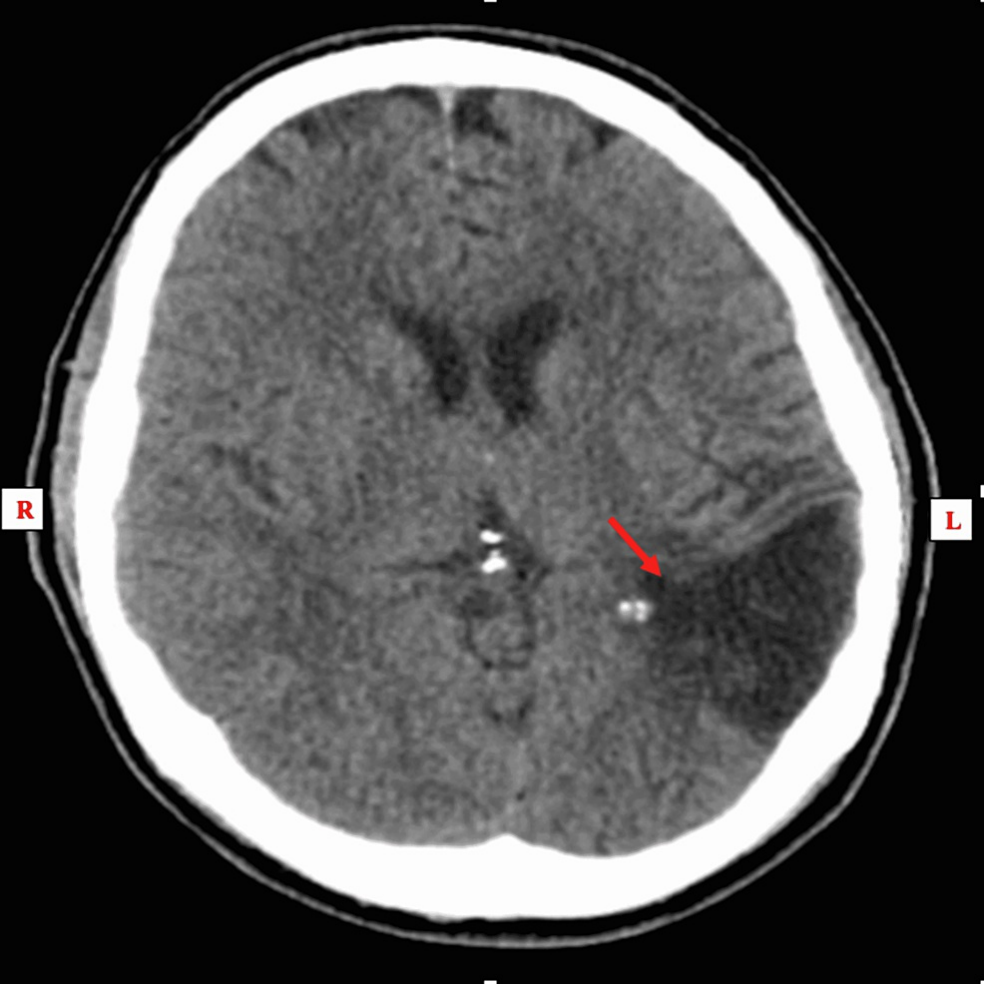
CT head without contrast (axial view); re-demonstration of encephalomalacia in the left temporoparietal lobe from the prior infarct
